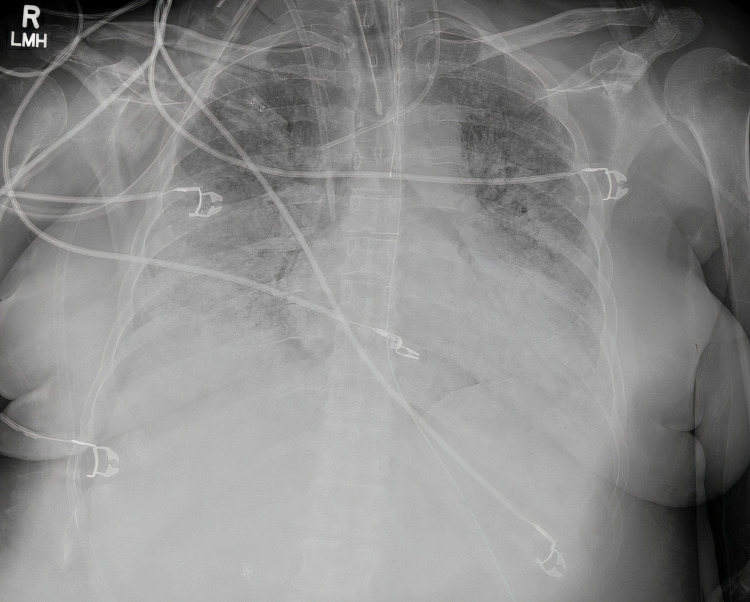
Initial chest x-ray obtained at tertiary care facility displaying bi-basilar opacities prominent within right lung fields.
